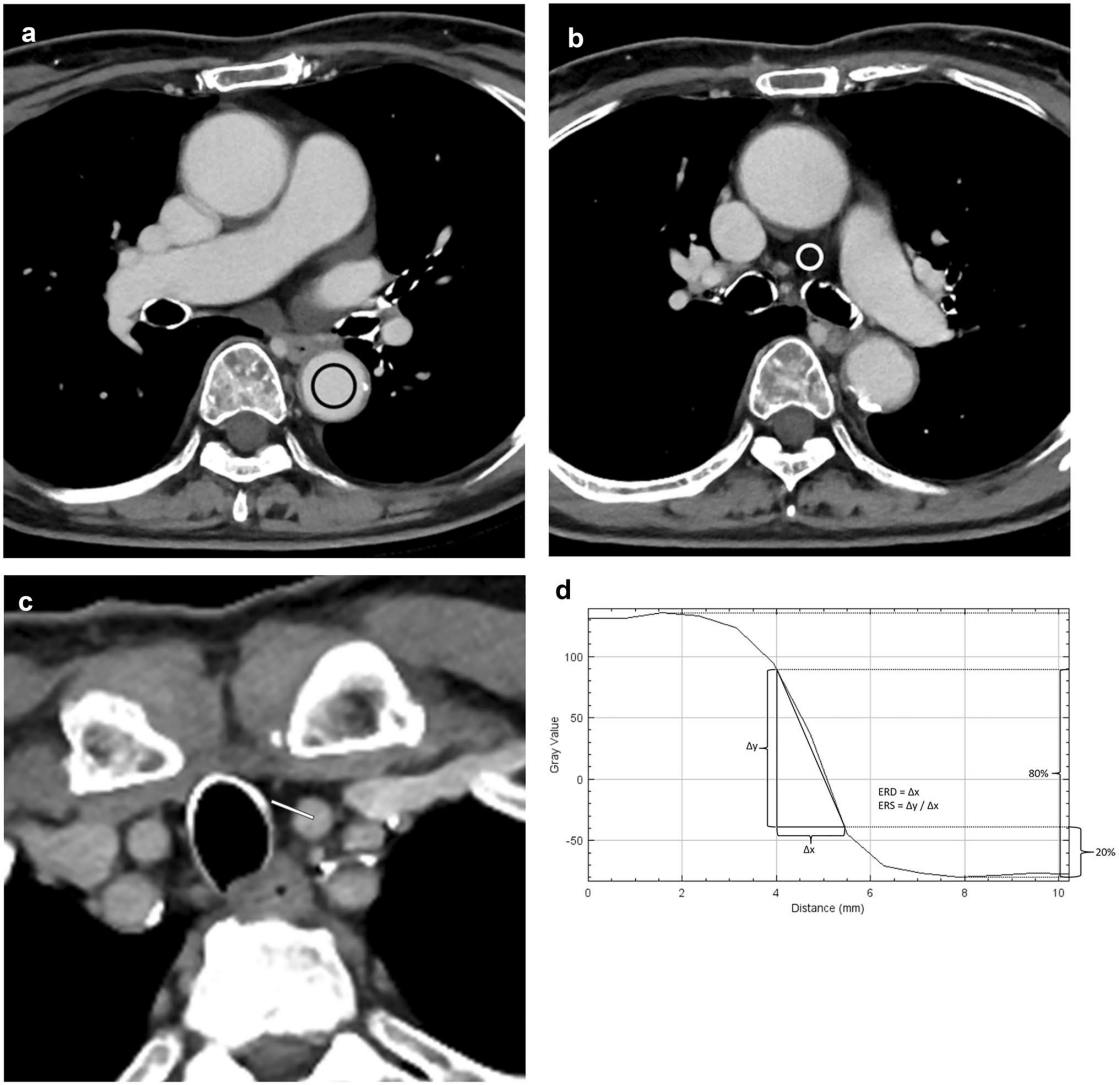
Circular or ovoid regions of interest were placed on (a) the aorta (black circle) and (b) mediastinal fat (white circle). A linear region of interest (white line) was placed transversely across the wall of the left common carotid artery in a rectangular manner (c). From the CT attenuation profile along the linear region of interest, edge rise distance (ERD) and edge rise slope (ERS) were calculated with setting the reference points on the curve with 20%–80% values (d)
